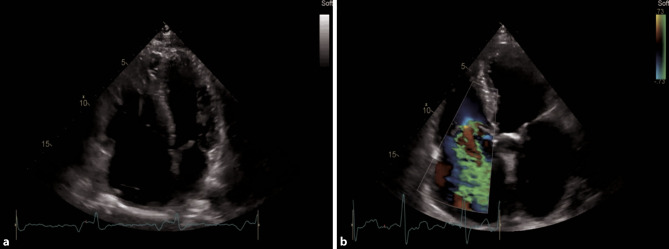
Pathologies of the right heart in an apical 4‑ChV. a RV dilatation due to myocarditis. b Severe tricuspid regurgitation
